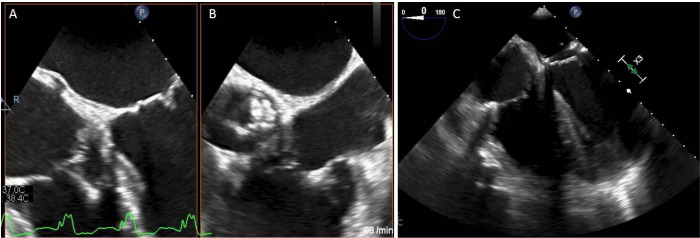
(A) Preoperative echocardiography showing RV lead with vegetation. (B) Echocardiographic image of the aortic valve with large endocarditis vegetation. (C) Intraprocedural transesophageal echocardiography (TEE) during lead extraction, showing complete removal of the RV lead and exclusion of pericardial effusion after lead extraction.
